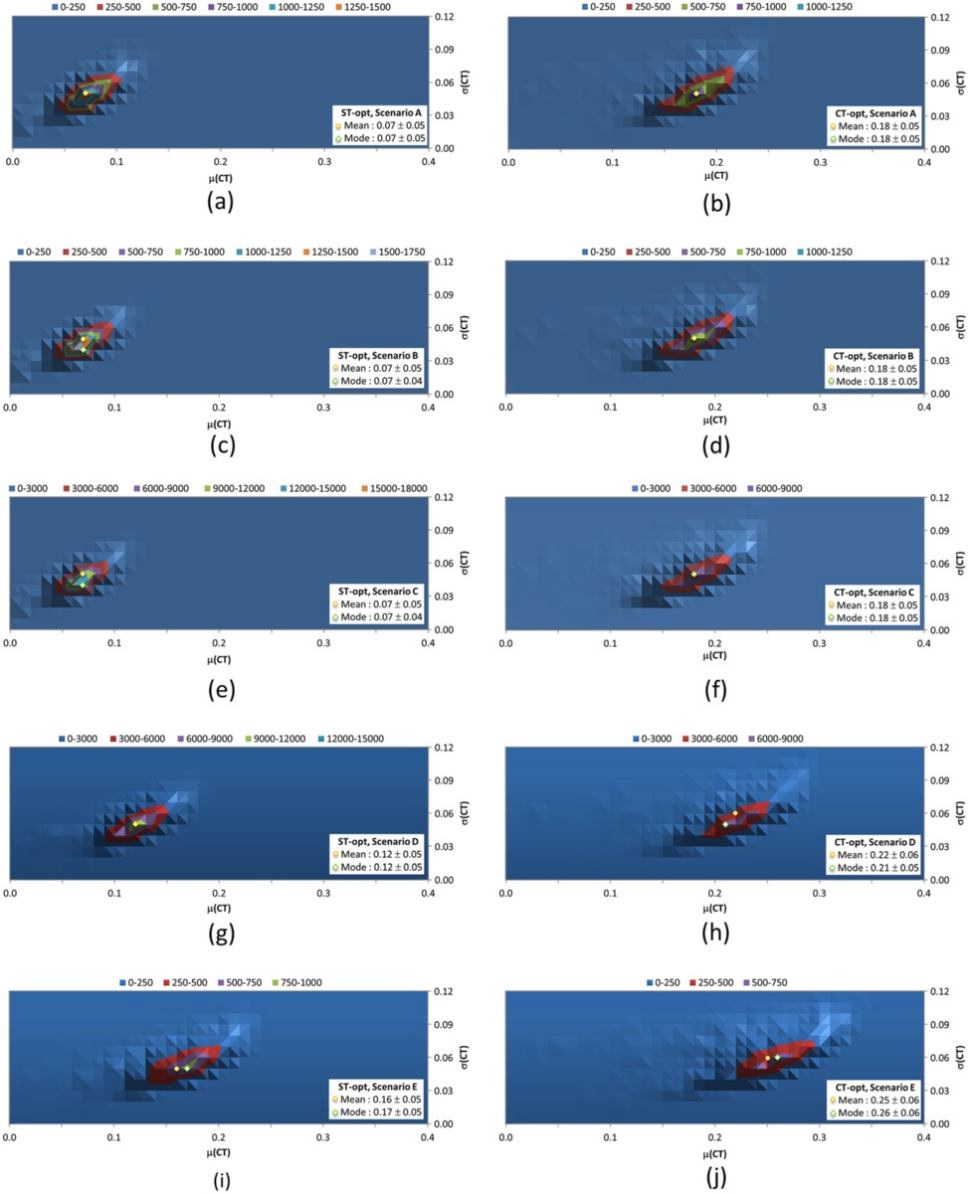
Average and standard deviation distributions for color-Tanimoto (CT), per “query”. Binned distributions in 0.01 increments of the average and standard deviation of the color-Tanimoto (CT) scores per query-type for the five similarity search scenarios tested (see Table 1): *Scenario A* [(a) and (b)], *Scenario B* [(c) and (d)], *Scenario C* [(e) and (f)], *Scenario D* [(g) and (h)], and *Scenario E* [(i) and (j)]. The left panels [(a), (c), (e), (g), and (i)] are for the ST-optimized CT scores, and the right panels [(b), (d), (f), (h), and (j)] are for the CT-optimized CT scores.
